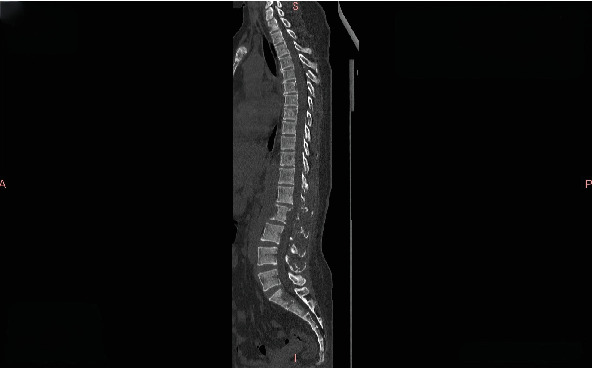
Thoracic and lumbar CT at the second progression in July 2020 showing multiple osteolytic destructions.
